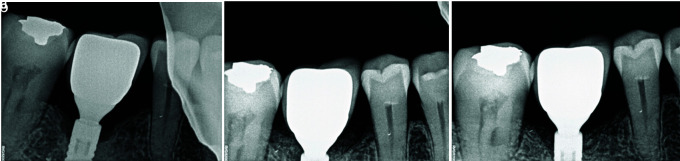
a: Pre-operative, b: immediate post-operative radiograph, c: radiograph at 6 months
